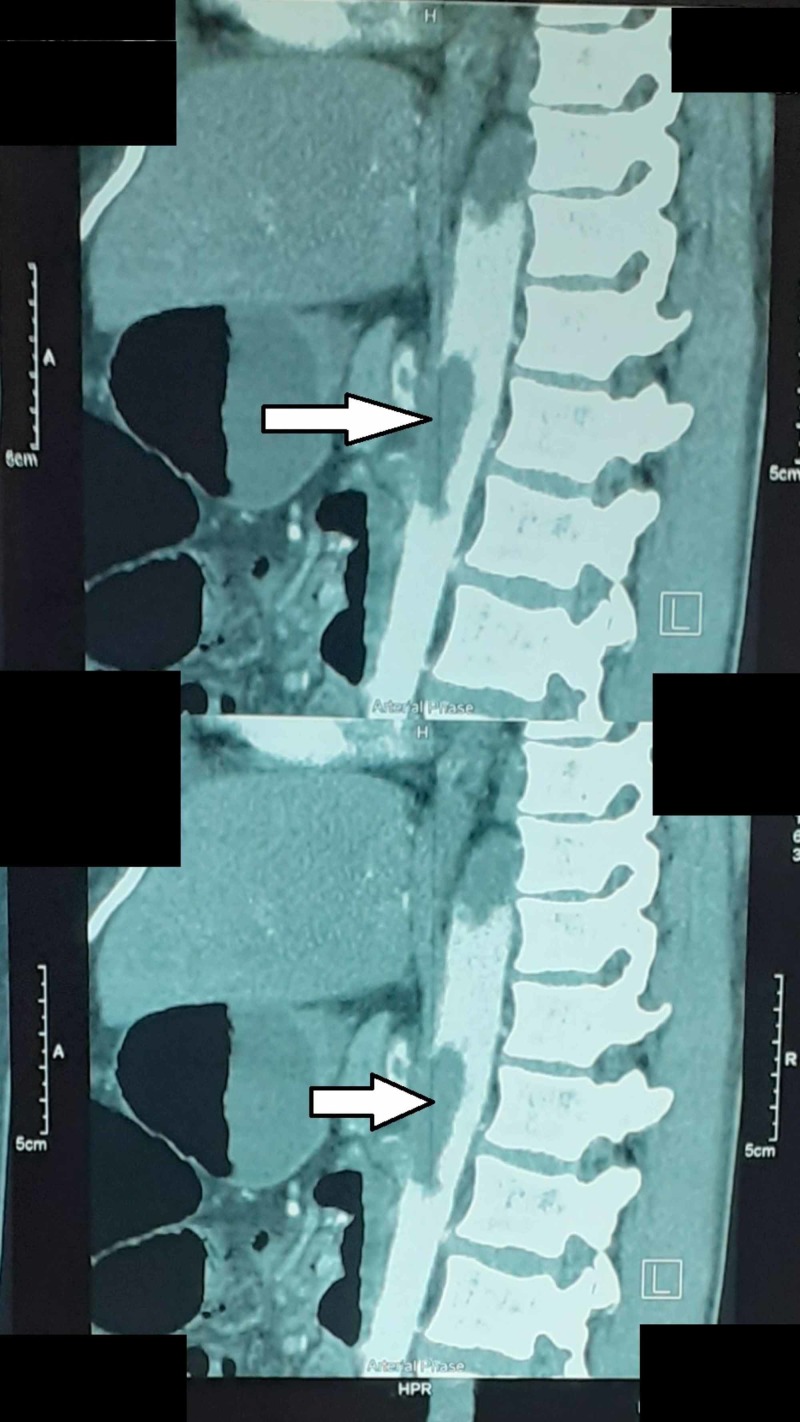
CTA showed aortic thrombus extending into the celiac axis and superior mesenteric artery CTA, computed tomography angiography
